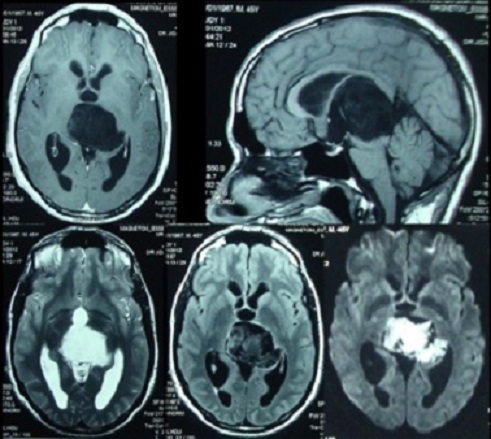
Preoperative MRI of a Pineal Epidermoid cyst. The MRI showed a lesion of the pineal region, hypointense T1-weighted with no contrast enhancement, hyperintense T2-weighted. Diffusion weighted images showed a bright at the pineal region with extension on supratentorial
